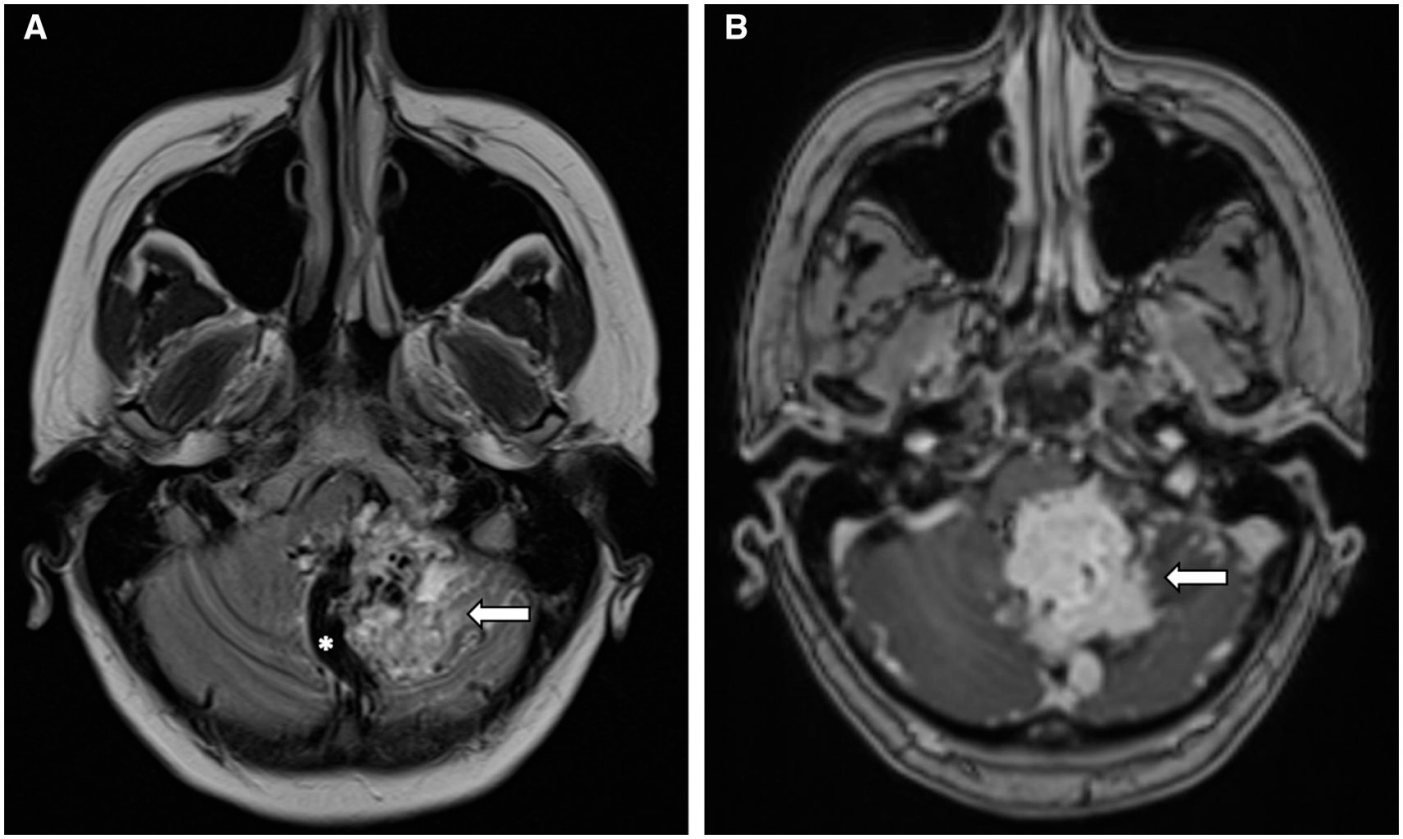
Cerebellar haemangioblastoma. (A) Axial T2-weighted MR image shows hyperintense multilobulated intraaxial mass (arrow) in the left cerebellar hemisphere, and serpiginous flow voids (asterisk). (B) Axial contrast-enhanced T1-weighted MR image shows solid avid enhancement (arrow).
